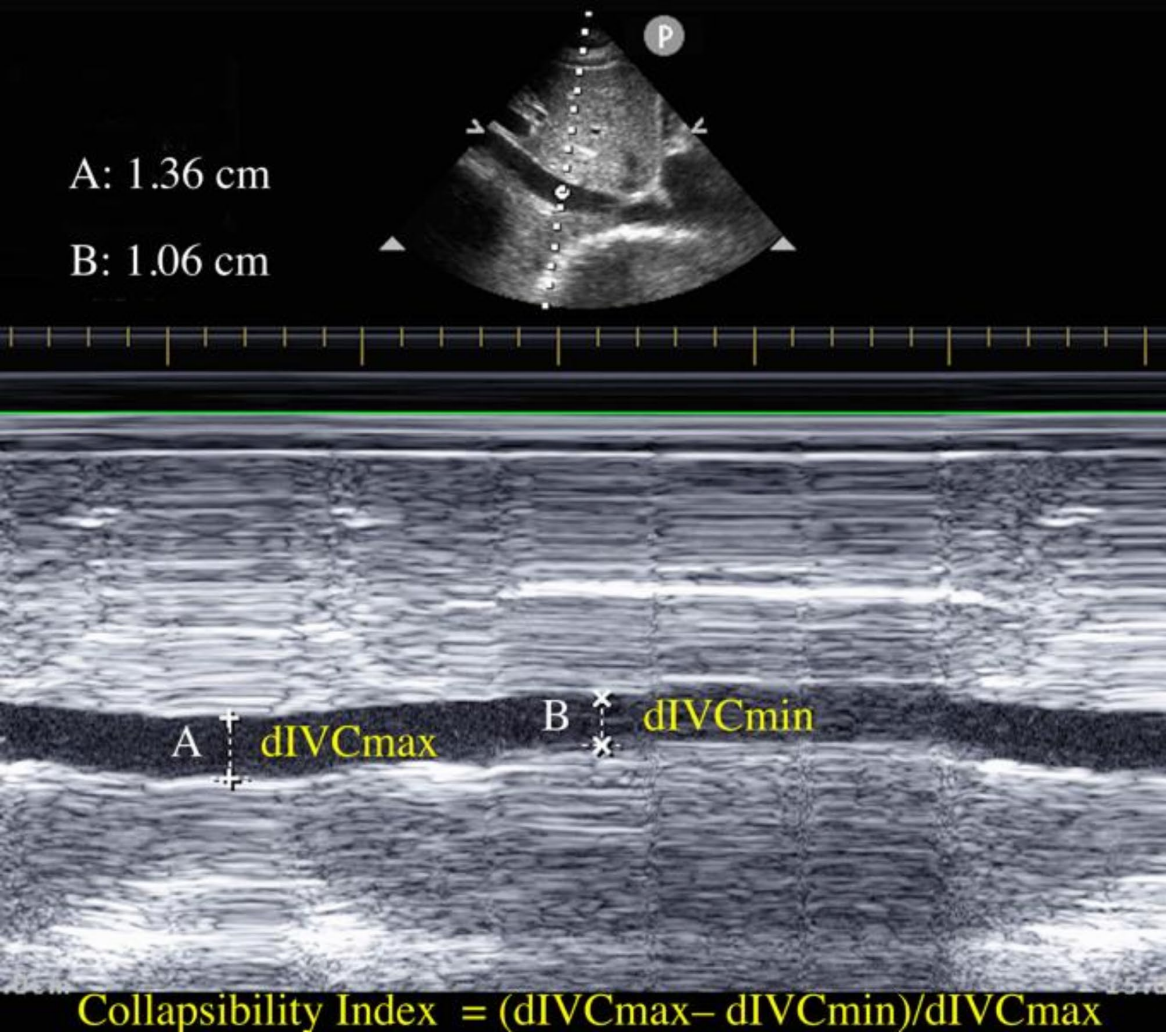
Ultrasound measurements of inferior vena cava (IVC) and calculation of collapsibility index. Panel above shows two-dimensional scan of the IVC, and panel below shows M-mode scan with respiratory variations in diameter. dIVCmax = maximum diameter of IVC; dIVCmax = minimum diameter of IVC
